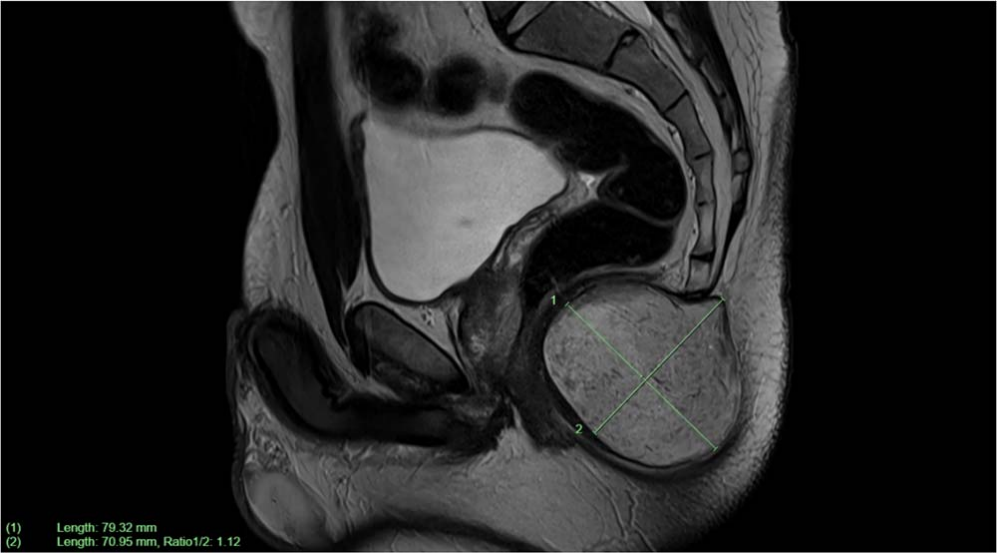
Sagittal plane MRI image of large subcutaneous cystic structure compressing on the anal canal—measuring 7.9 × 7.1 cm in size.
